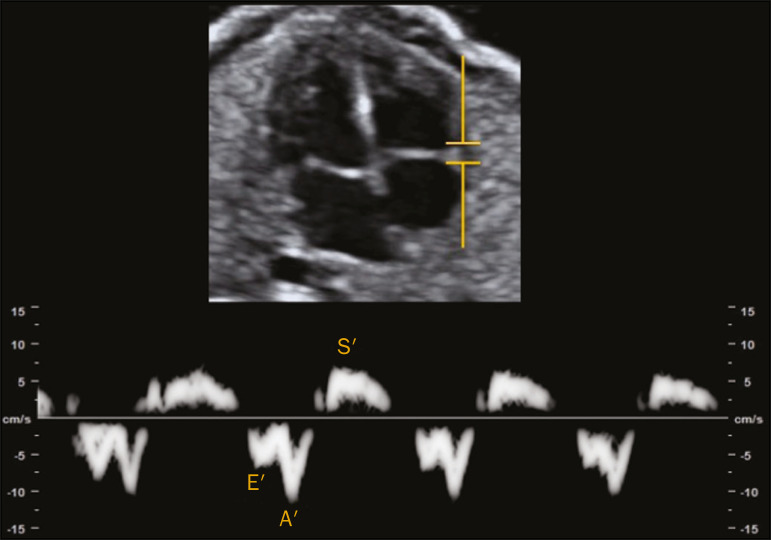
Peak velocity obtained by spectral tissue Doppler in the right annulus of the fetal heart.
